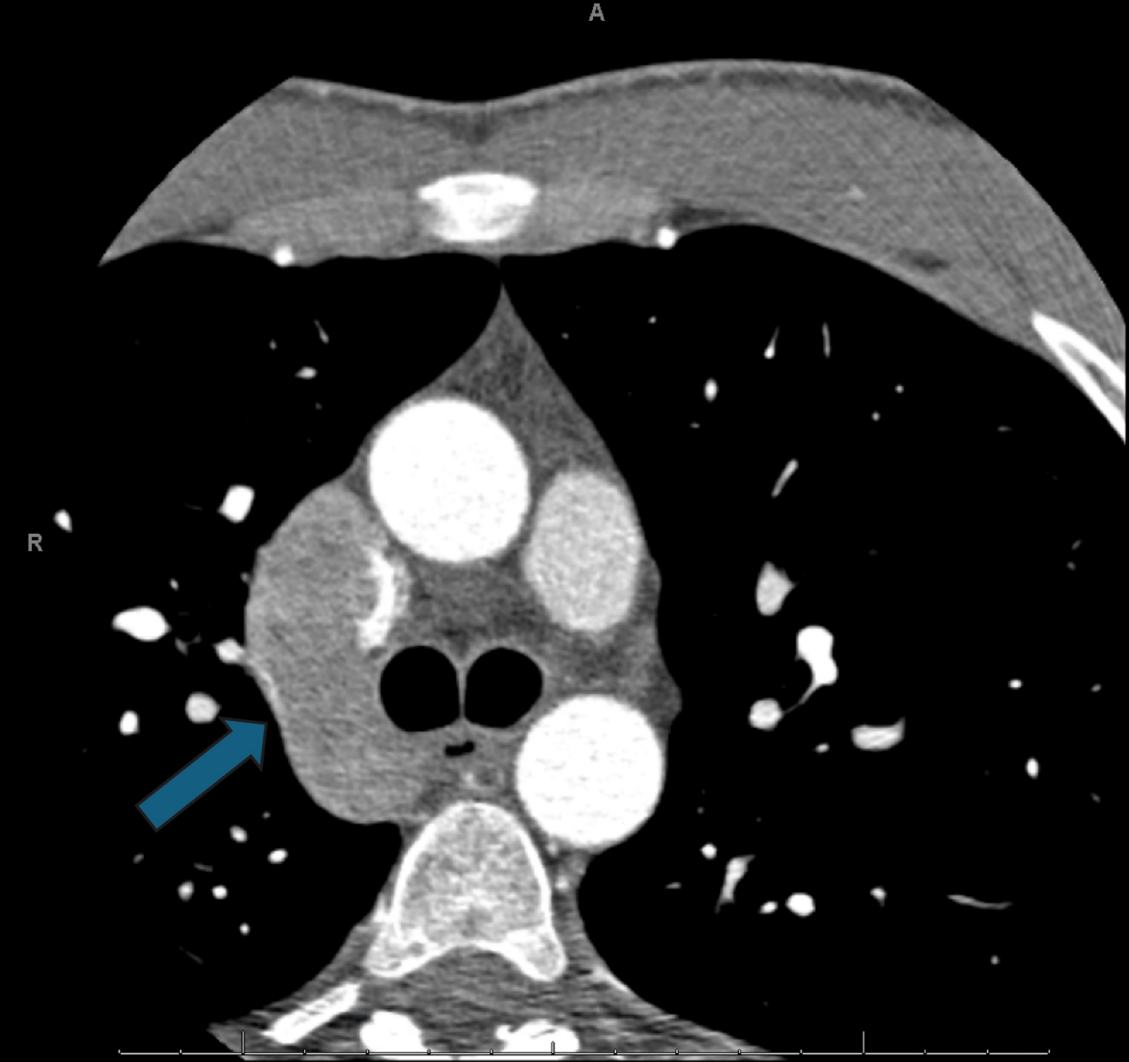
CT coronary angiogram: axial view at the thorax level, showing the distended azygous vein The blue arrow shows a dilated azygous vein, which ultimately connects to the superior vena cava. This distension suggests the azygos vein compensated for the interrupted IVC, carrying increased venous flow, which impacted the ability to advance the catheter into the right atrium via the transvenous route. These imaging findings were critical in guiding the decision to attempt a retrograde aortic approach for ablation, as the abnormal venous anatomy complicated standard access.
